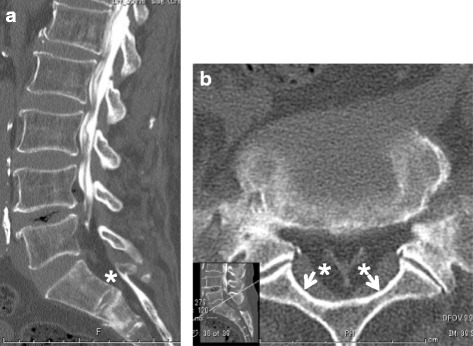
Computed tomography myelogram. a Reconstructed sagittal view, b axial view. Saucerization of the laminae (arrows) with a homogeneous hypodense epidural mass (asterisks) is shown at the L5-S1 level
